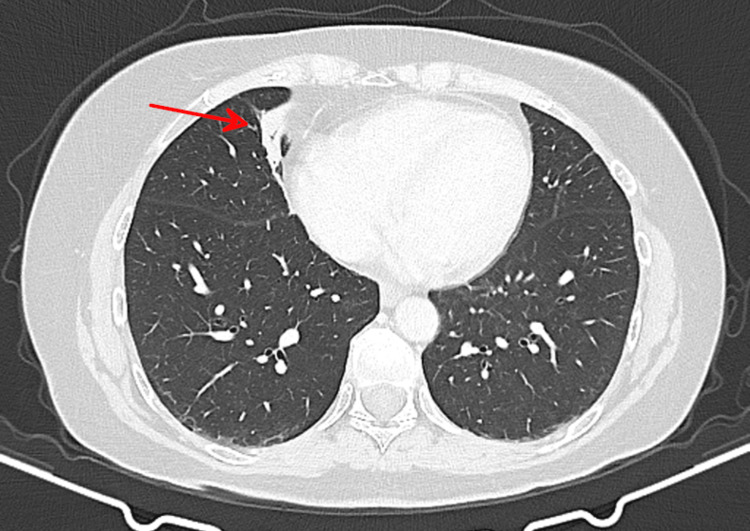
Baseline chest CT Subsegmental band-like atelectasis in the right middle lobe (red arrow). No suspicious pulmonary nodules or masses are identified. CT: computed tomography
